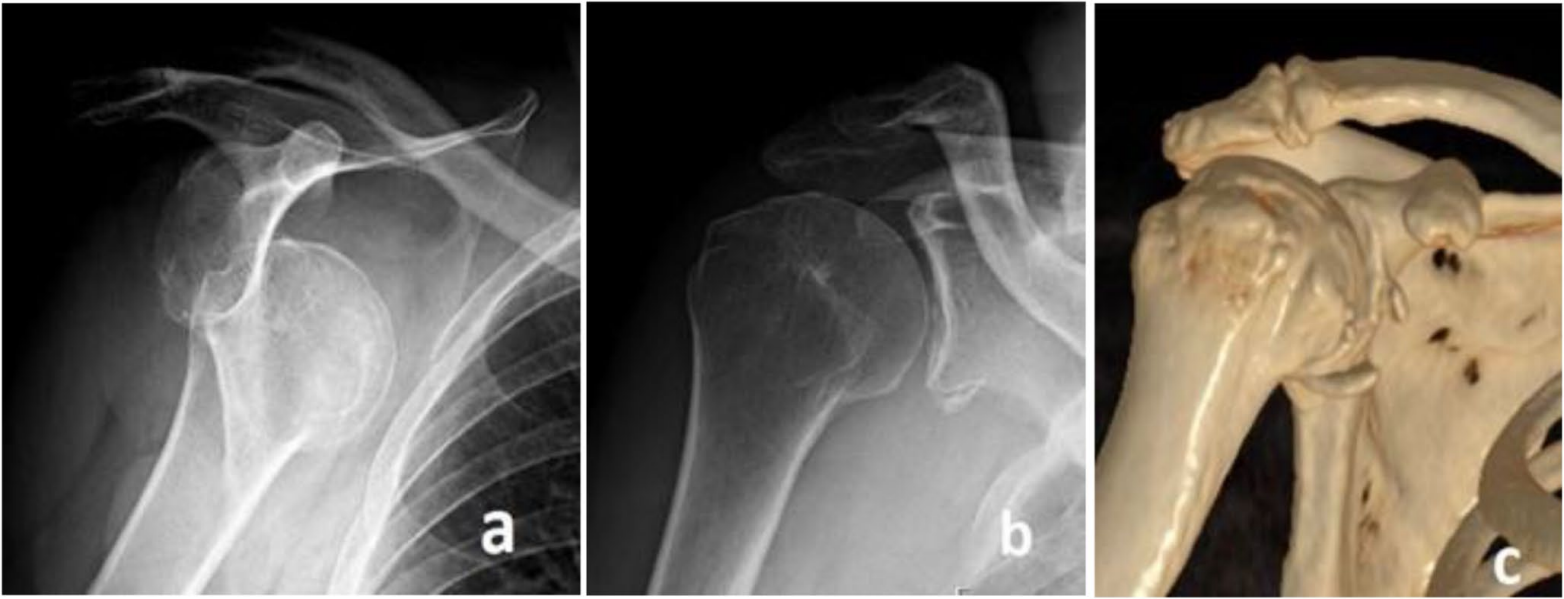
a: dislocated shoulder x-ray, b: post reduction Grashey view, c: 3D reconstruction CT scan
